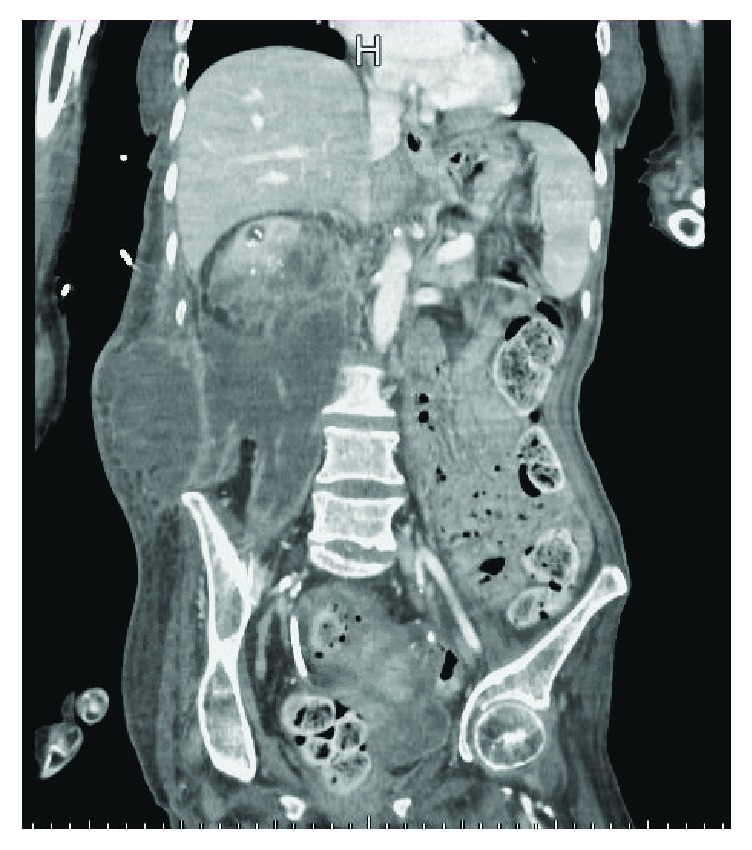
Coronal section of a CT scan if the abdomen/pelvis with IV contrast revealing severe hydronephrotic right kidney with accompany urinoma in the retroperitoneum.
